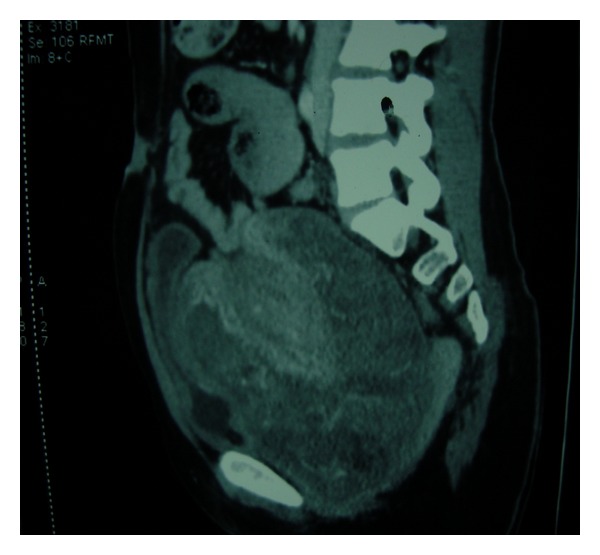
Sagital section computed tomography showing the lesion compressing the bladder anteriorly and the rectum posteriorly maintaining the intervening fat planes.
